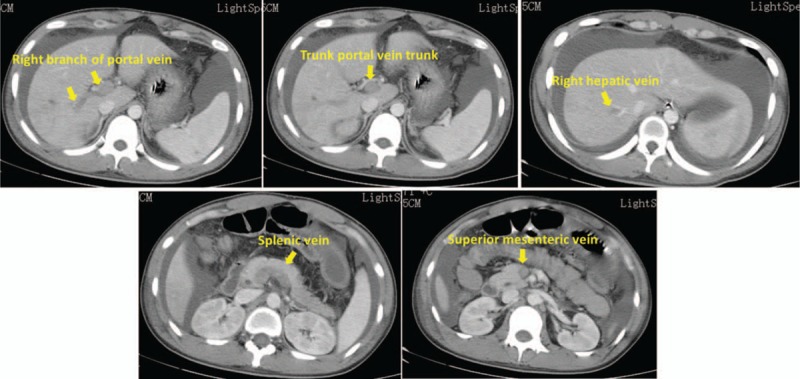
Whole abdominal enhanced computed tomography scan on July 21, 2017. The arrow in the figure refers to veins with extensive venous filling defects in the contrast-enhanced scan during the venous phase, suggesting venous thrombosis.
